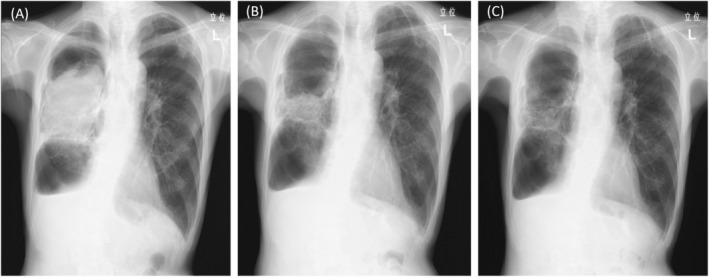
(A) Chest radiograph of anteroposterior view on the first hospital day. (B) Chest radiograph at 5 months after treatment initiation indicated that fugus ball had decreased in size. (C) Chest radiograph at 6 months after treatment initiation indicated that fugus ball had nearly resolved.
